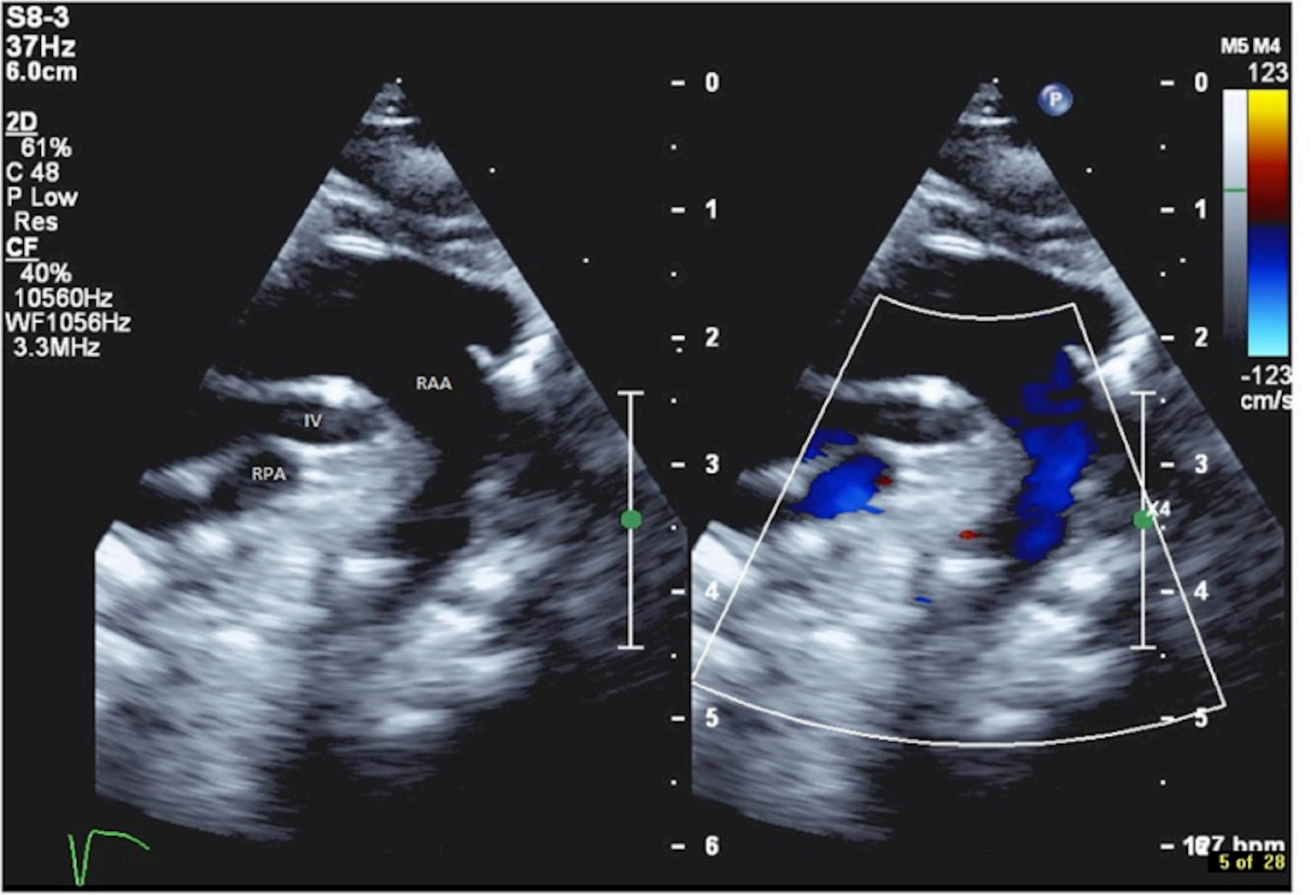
Echocardiography showing the retro-aortic position of the innominate vein (IV), positioned between the right aortic arch (RAA) and the right pulmonary artery (RPA).
